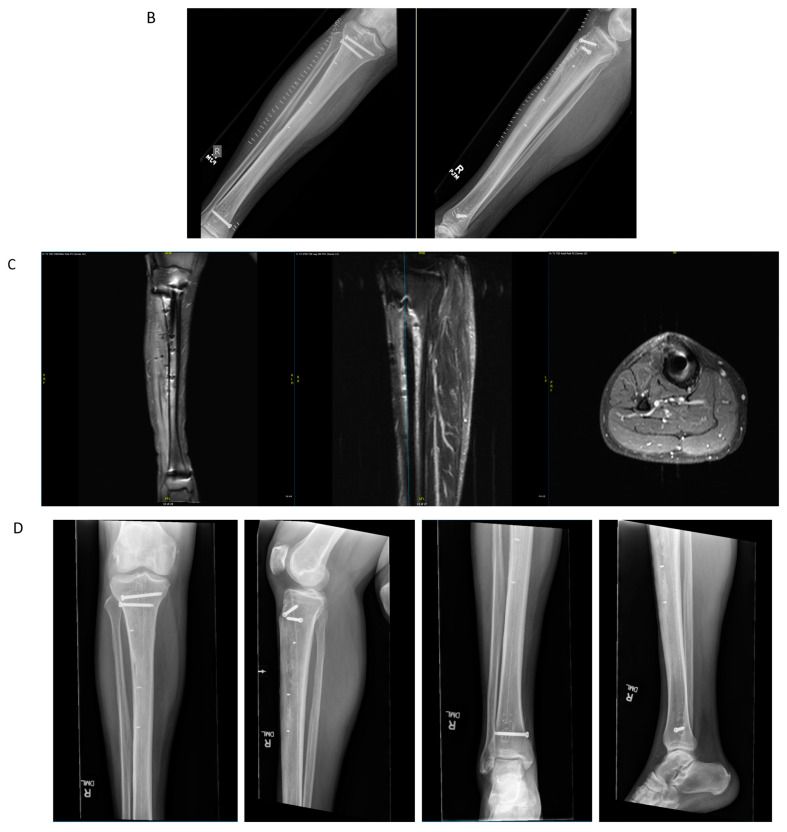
(A) Pre-operative T1 post-contrast, fat-saturation coronal; T2 fat-suppression sagittal; and T1 post-contrast, fat-saturation axial magnetic resonance images of a right tibia demonstrating a myxoid liposarcoma. (B) Post-operative radiographs demonstrating interval placement of an intramedullary carbon fiber nail. Note is made of a cortical defect in the tibia to allow for adequate resection margins; as such, a carbon fiber was placed for fracture prophylaxis. (C) The 9-month post-operative T1 post-contrast, fat-saturation coronal; T2 fat-suppression sagittal; and T1 post-contrast, fat-saturation axial magnetic resonance images demonstrating interval resection of the myxoid liposarcoma. Reduced artifact from carbon fiber nail facilitated visualization of the resection bed for monitoring of local recurrence. (D) The 20-month post-operative radiographs of the right tibia with intramedullary carbon fiber nail in place and healed cortical defect from prior resection margin.
